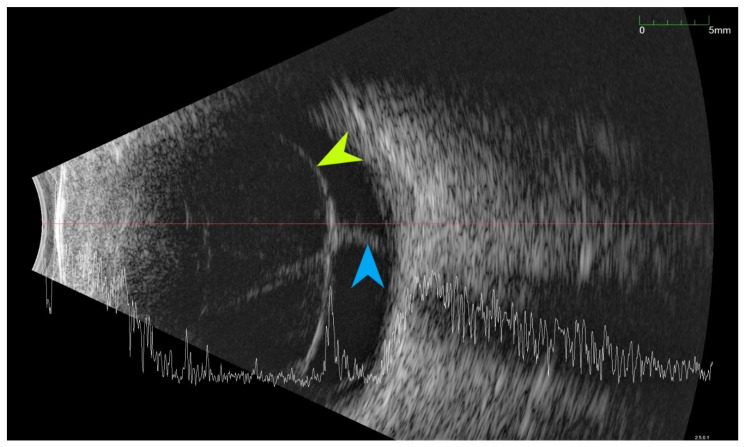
Ultrasound examination showed hyperechoic densities and point tractions on the retina (blue arrowhead). Densities forms strands and membranes with reduced mobility (green arrowhead). In order to confirm that the retina is not detached, the A-scan was superimposed over B-scan.
